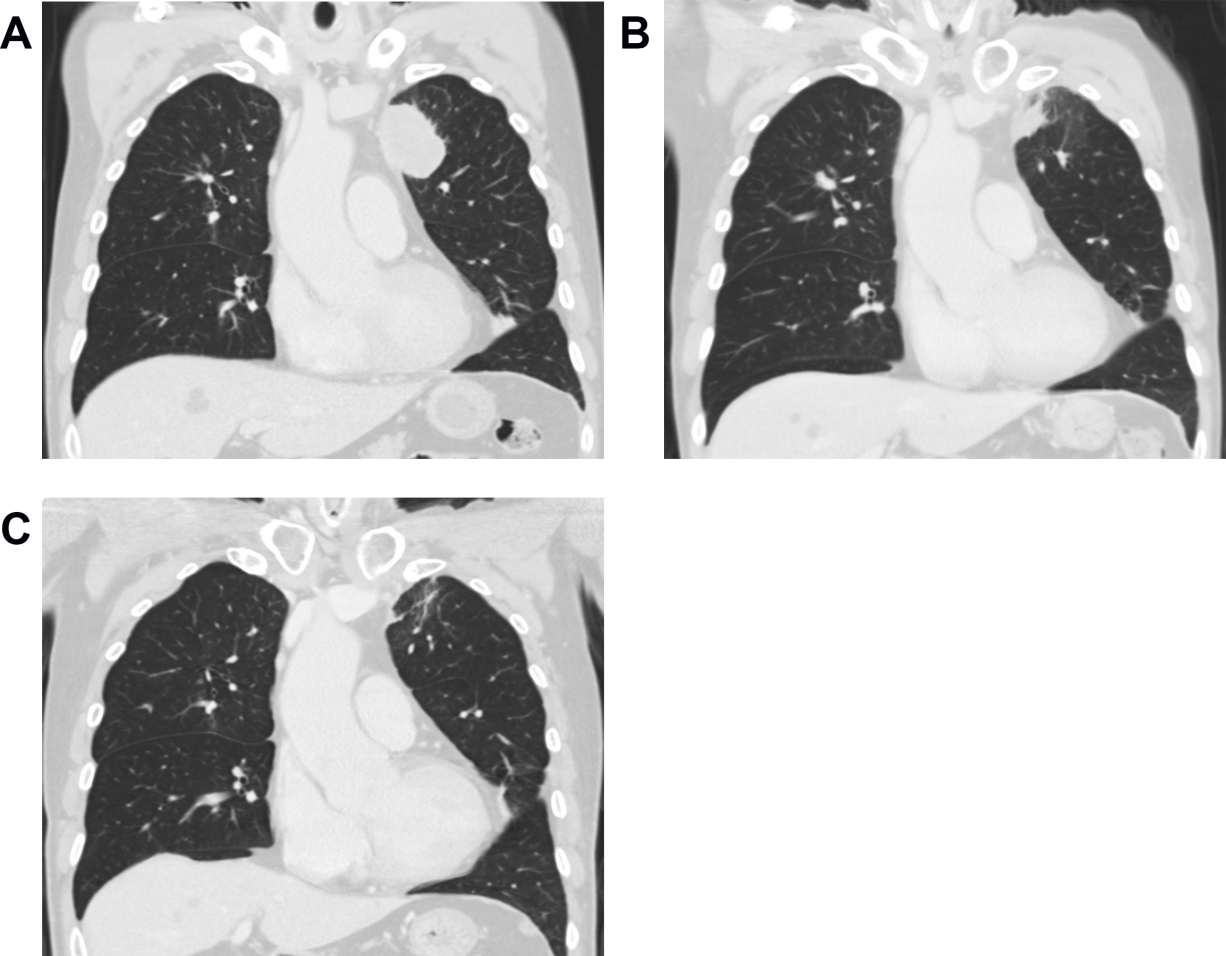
One patient presented with complete remission of thoracic tumor after radiotherapy. This 66-year-old man had initial lung to lung metastasis, and the disease was controlled by chemotherapy with docetaxel and cisplatin. However, tumors at left upper lobe and mediastinum progressed 5 months after diagnosis. Mediastinal lesions improved after chemotherapy with gemcitabine and vinorelbine, but left upper lung tumor remained stationary. Thoracic radiotherapy (RT) with 55 Gy in 25 fractions was applied to left lung tumor and 45 Gy in 25 fractions to mediastinal lymphatics using IMRT. Complete remission of thoracic lesions was achieved five months after completing RT. Only grade 1 radiation pneumonitis was noted. He remained disease-free 17 months after RT without systemic chemotherapy. (A) Chest CT scan before thoracic RT. (B) Chest CT scan two months after thoracic RT. (C) Chest CT scan five months after thoracic RT.
